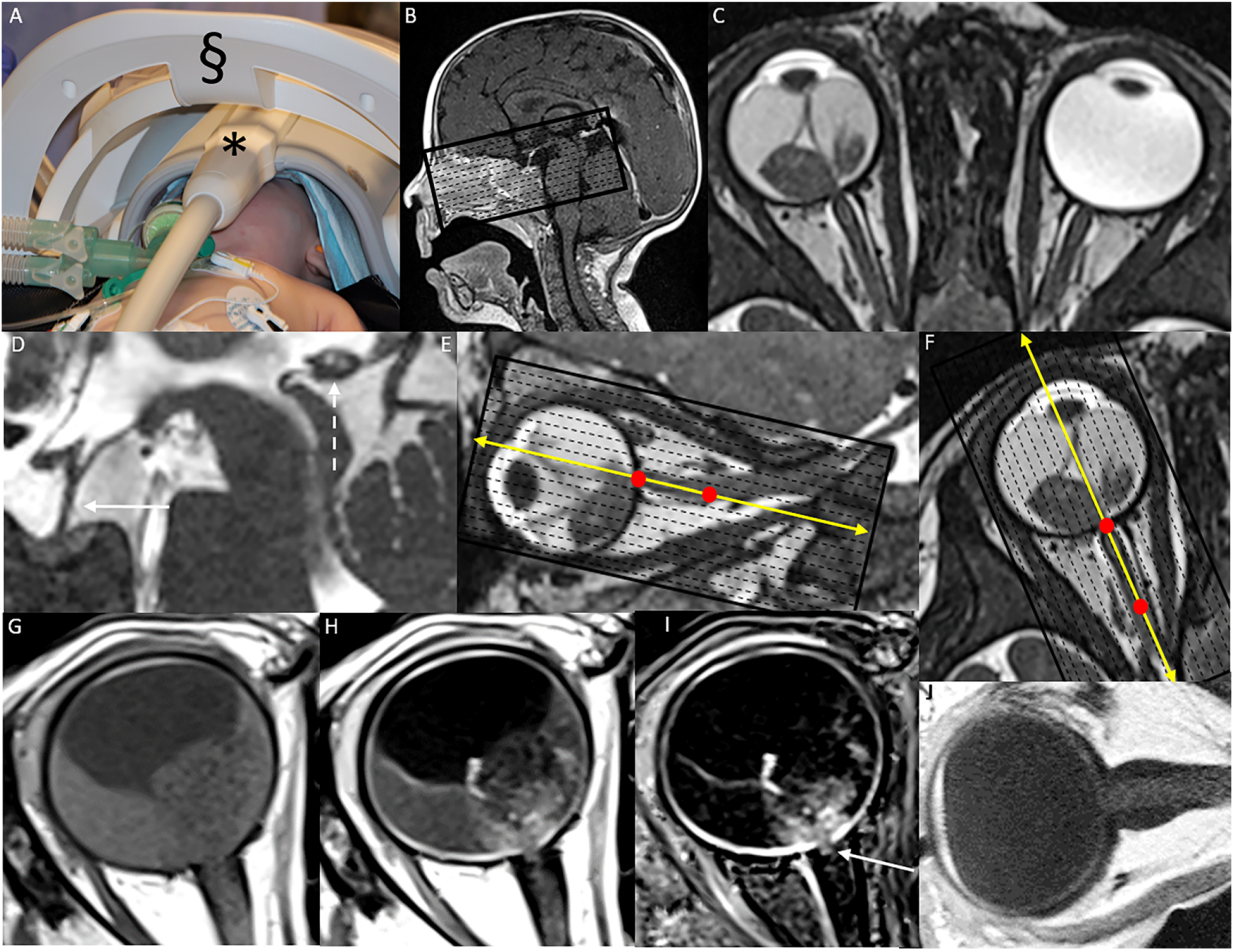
Placement of an infant in an MRI machine and 3D heavily T2-weighted sequence and 2D T1-weighted SE images centered on the orbits: A The infant, under general anesthesia, is positioned with an orbit surface coil (asterisk) and a head coil (section) for imaging in a 1.5-T MRI machine. B A scout image shows the selected area of interest, centered on the orbits and including the pineal region. C Axial heavily T2-weighted MR image, centered on the orbits and oriented in the plane of the optic nerve, revealing an exophytic intraocular mass in the right eye, associated with complete retinal detachment. D Sagittal reconstruction showing a normal suprasellar region (arrow) and a normal pineal gland (dashed arrow). E The axial and F sagittal obliques T1 sequences are planned along the orientation of the retrobulbar portion of the optic nerve (red dots), based on a reconstructed sagittal and axial plane of the 3D high-resolution heavily T2-weighted images. The central slice must be positioned precisely in the middle of the nerve (yellow arrows). G Axial T1-weighted sequence before contrast and H axial T1-weighted sequence after contrast, both oriented along the optic nerve axis. I Subtraction of the pre- and post-contrast T1-weighted sequences reveals subtle contrast enhancement (arrow), indicating postlaminar optic nerve infiltration. Although Dixon sequences can also be used, they have the drawback of increased acquisition time due to fat saturation. J Sagittal T1-weighted sequence after contrast, oriented along the optic nerve axis
